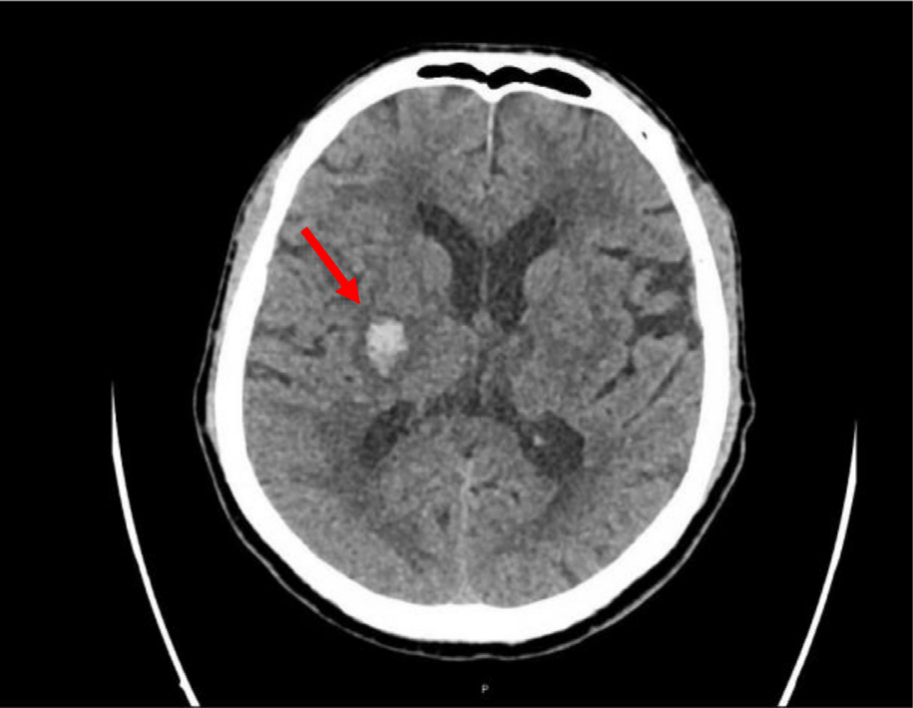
Unenhanced CT brain findings: [Red arrow] right basal ganglia hyper-density suggestive of acute parenchymal bleed measuring approximately 23 × 16 × 12 mm. Left basal ganglia Ill-defined hypodensity likely represents sequela of old insult. Bilateral periventricular/white matter hypodensities suggestive of microangiopathic changes. No significant midline shift or hydrocephalus. Preserved basal cisterns.
